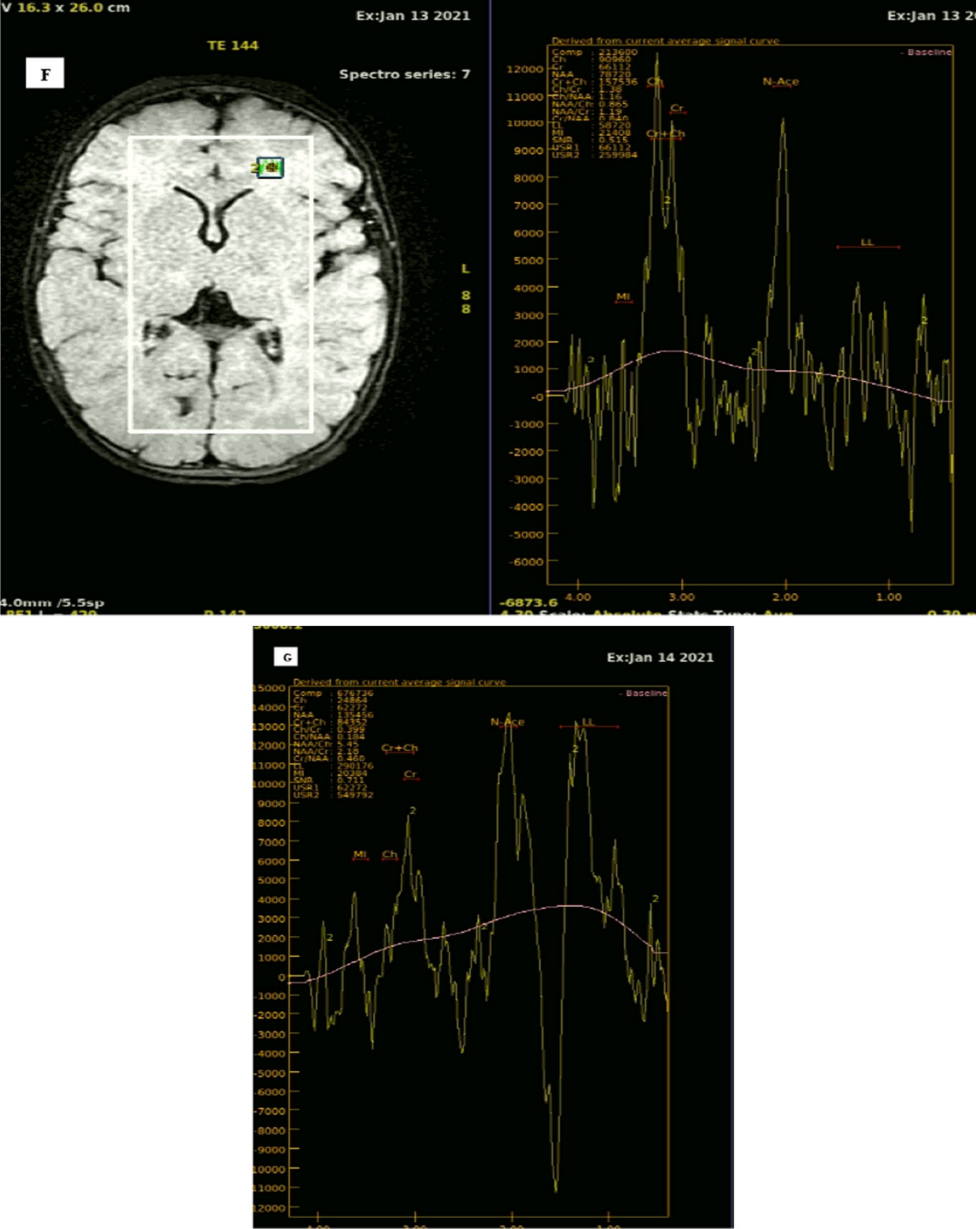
A 49-year-old man with positive PCR for COVID-19 presented with fever, dyspnoea, after 10 days the patient came with convulsions. A Axial FLAIR showing non-confluent multifocal white matter hyper intense lesions are seen in both fronto-temporal lobes and periventricular regions, B axial T2WI, showing hyperintense signal of the previously mentioned areas of altered signal, C coronal T2WI, D DWI (*b*-value 1000) showing free diffusion of the areas of altered signal, E long TE 144 (at right frontal region), F long TE 144 (at left frontal region); both show mild increase level of choline with mild decrease in NAA peak, G short TE35 show high bifid peak of lipids and lactate…. Diagnosed as COVID induced demyelination
